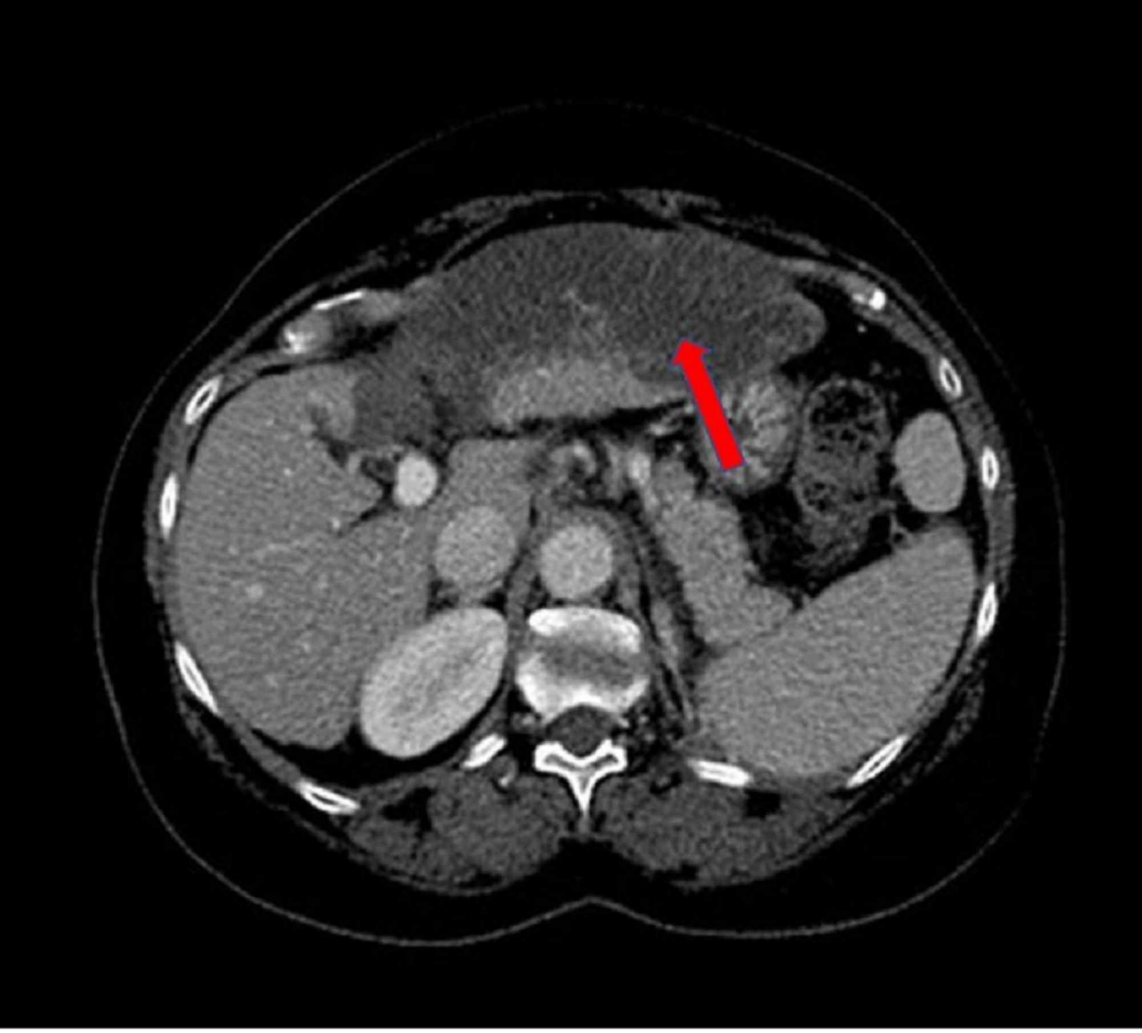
CT angiogram of the abdomen revealing thrombosis of the left branch of the hepatic artery with hepatic infarct (red arrow).
